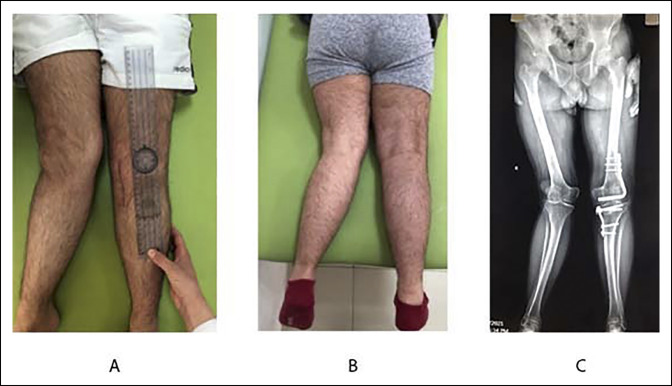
A and B, Photographs showing the clinical difference between the corrected left side and the untreated right side. C, The 7-month postoperative anterior-posterior radiograph showing knee deformity correction and complete healing of osteotomized bones.
